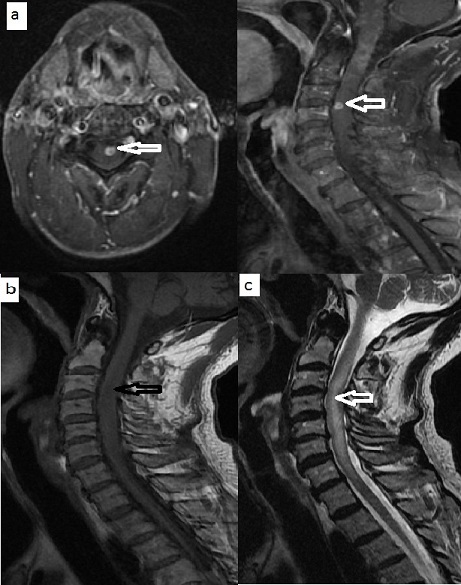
A) sagittal MRI and axial T1 sequence with gadolinium injection: annular contrast enhancement zone at the anterior portion of the cervical spinal cord opposite C4-C5 (arrow); B) Sagittal MRI T1 sequence: isointense nodule (arrow); C) Sagittal MRI T2 sequence: fusiform aspect of the spinal cord resulting in an intramedullary edema (arrow)
